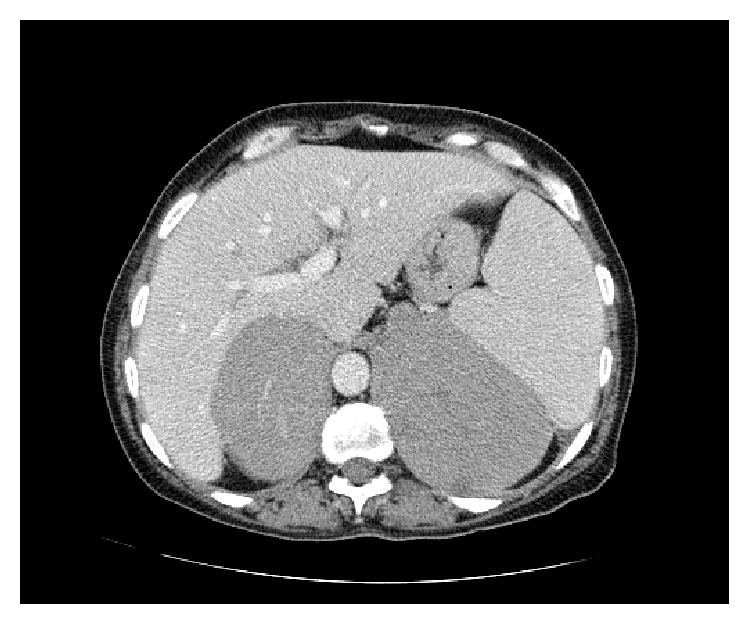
Spiral 64-slice CT scan obtained following intravenous contrast (100 mL Iohexol) in portovenous phase demonstrates marked, homogenous bilateral adrenal gland enlargement with no evidence of disease elsewhere.
